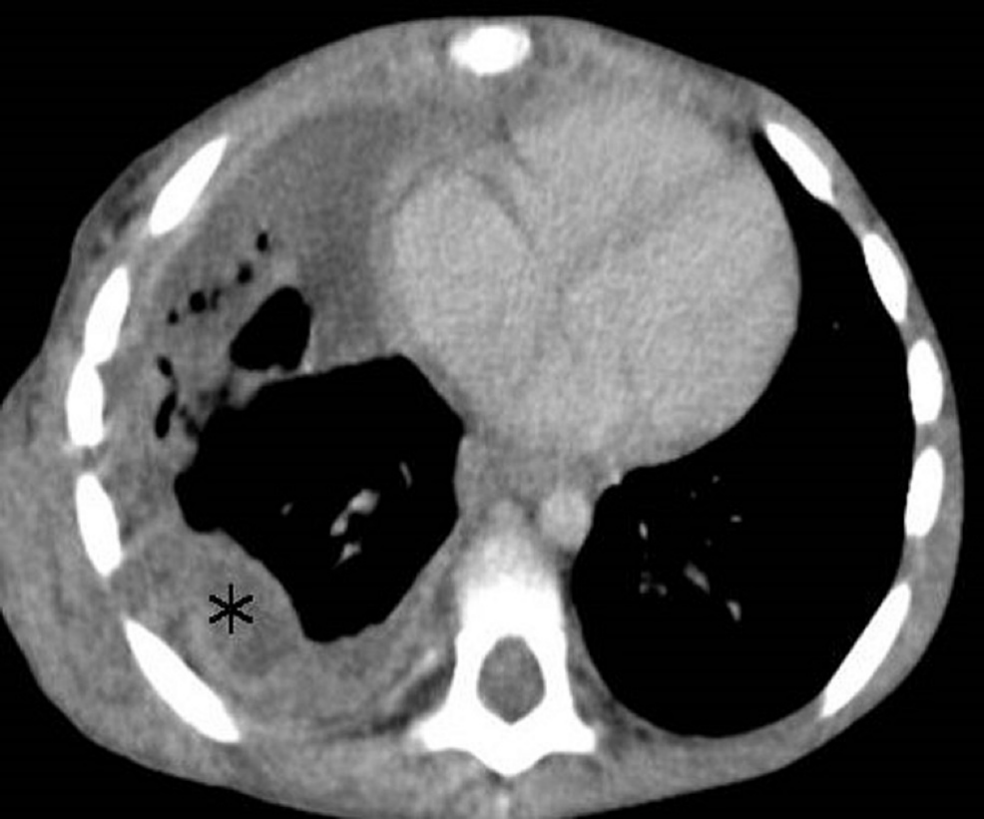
Thoracic CT scan Axial CT showing an irregular posterior and right lateral basal pleural (star) thickening suggestive of a recurrence.
